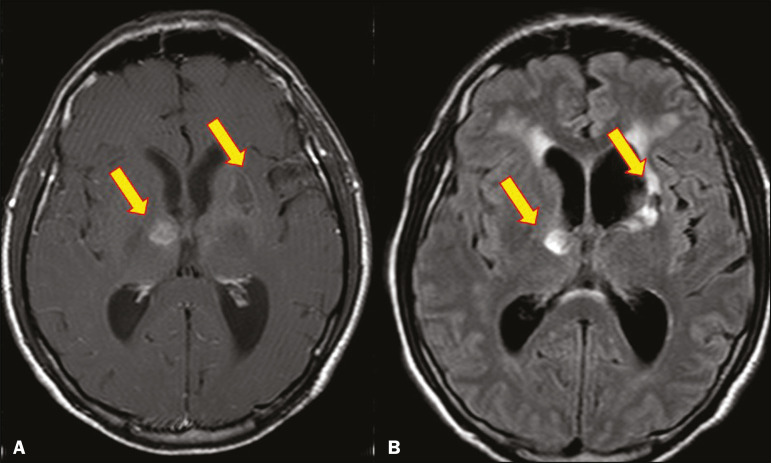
Infarcts by vasculitis secondary to histoplasmosis in a 38-yearold, previously healthy, patient who had lived in caves full of bats in Palestine and presented with progressive dementia. Biopsy demonstrated vasculitis secondary to histoplasmosis of the CNS. A,B: Contrast-enhanced FLAIR MRI sequences showing hyperintense lesions in the basal ganglia, consistent with subacute stroke.
